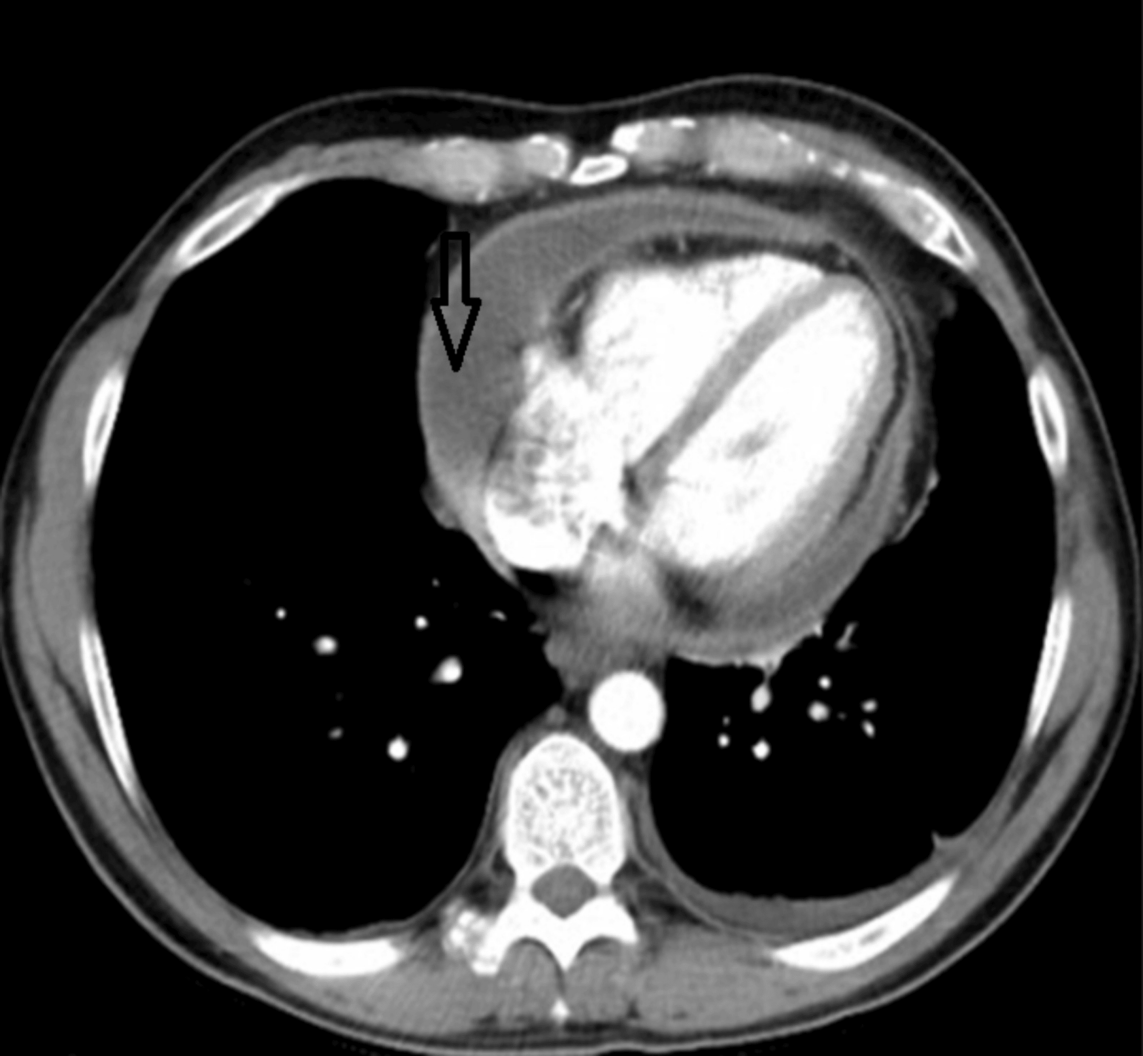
Chest CT scan (axial) The image shows pericardial fluid with a maximum size of 3.04 cm.
